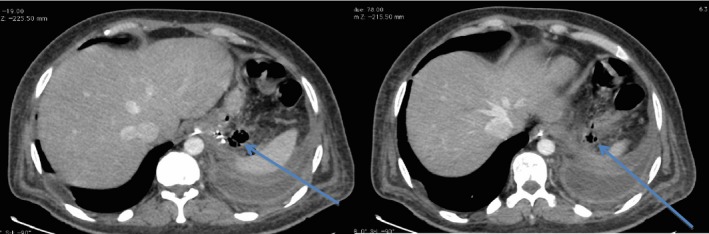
CT scan 10 days after admission with persistence of gastro‐colic fistula with evident air bubbles at the site of fistula (indicated by arrows).
